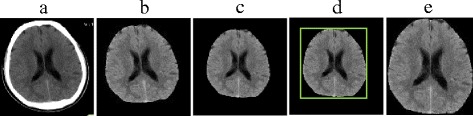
Example of normalizing a brain CT image a Original image *I*. b Image with the extracted intracranial portion. c Rotated image. d Image with its vertical external matrix. e Normalized grayscale image *GI*.
